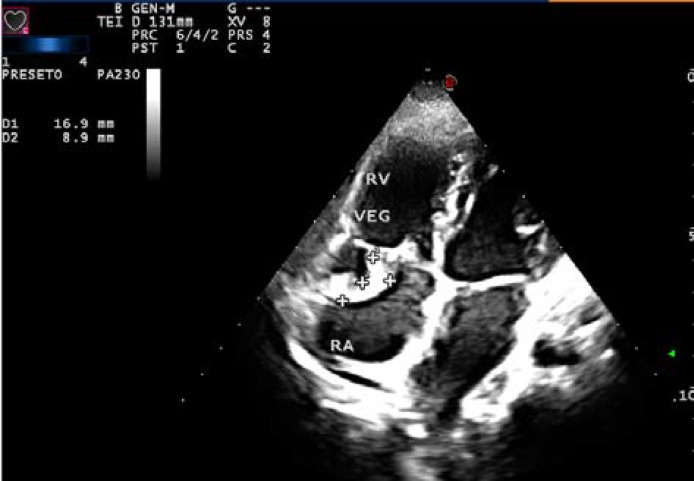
2D Echocardiogram of patient with vegetation in the anterior leaflet of tricuspid valve caused by indwelling port catheter
